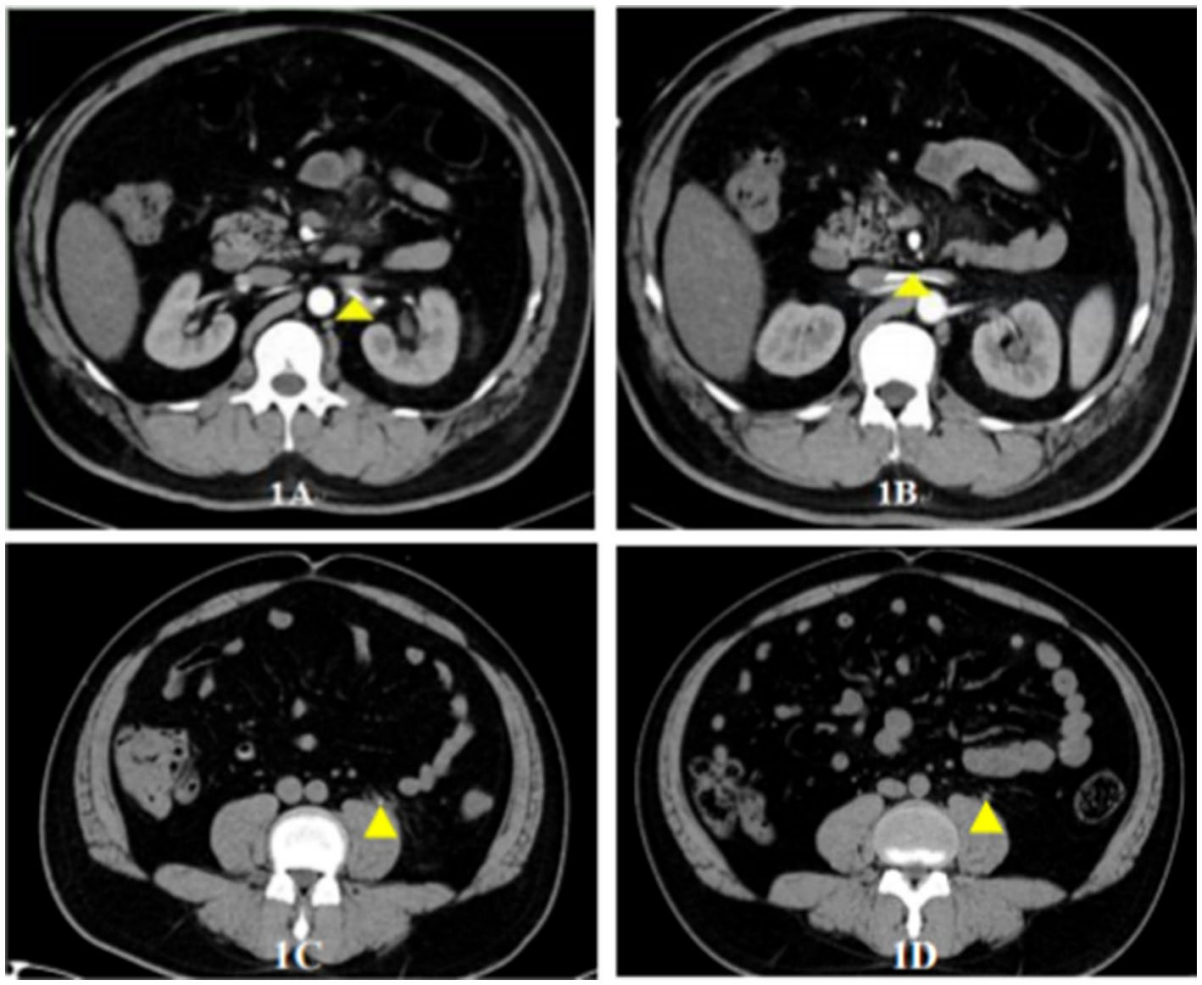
Radiographic documentation of double-J stent migration and associated urological changes. (A) Coronal CT reconstruction: Annotated marker (yellow triangle) demonstrates the aberrant trajectory of the double-J stent traversing from the left ureter into the left renal vein (Supplementary Video P1). (B) 3D vascular reconstruction: Sequential migration pathway visualized, showing stent progression from the left renal vein to the inferior vena cava (yellow triangular markers). (C) Axial CT at stent removal: Concurrent left ureteral calculus identified at the ureteropelvic junction (yellow annotation). (D) Follow-up CT at 2-week post-removal: Complete resolution of previously documented ureteral calculus, confirming stone elimination (yellow marker indicates original stone location).
